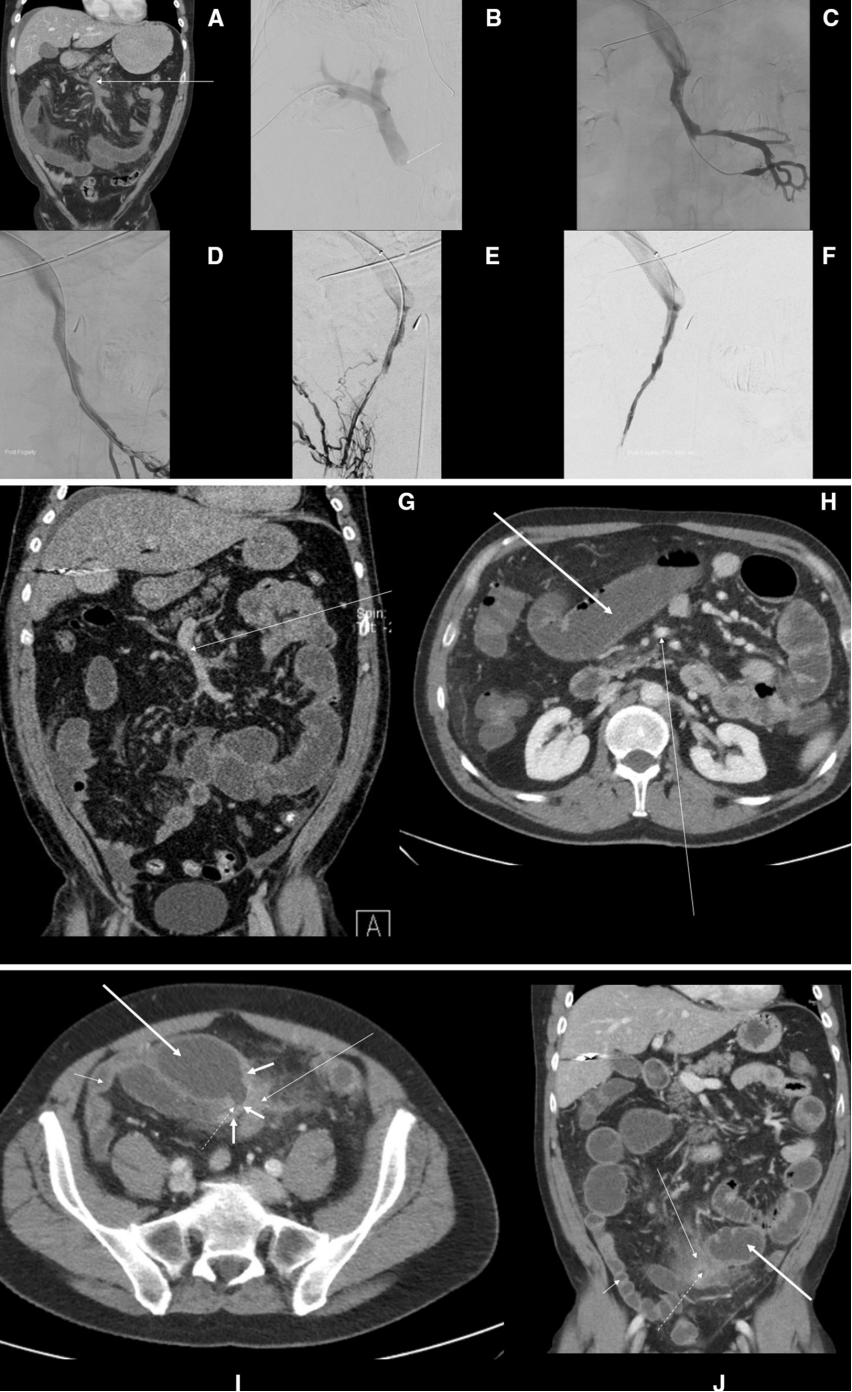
Endovascular therapy of mesenteric venous thrombosis after failure of anticoagulation treatment. A 53-year-old man with history of 3 months of anticoagulation treatment for deep venous thrombosis in the lower leg and Factor V Leiden mutation in the homozygous form. The patient fell ill with acute abdominal pain, and CT diagnosed an extensive MVT (a, arrow). He underwent transhepatic puncture and access to the portal vein. Venography showed total occlusion of the superior mesenteric vein (SMV) (b, arrow). Mechanical thrombectomy with an AngioJet® device (MEDRAD, Warrendale, Pennsylvania, USA) and endovascular Fogarty catheter thrombectomy were carried out, followed by thrombolysis with recombinant tissue plasminogen activator (rtPA) into the branches of the SMV and superior mesenteric artery. After a total dose of 25 mg rtPA over 25 h, improved flow in the SMV was noted. Endovascular rethrombectomy with a Fogarty catheter was performed owing to residual clots in the SMV branches (c–f). CT venography before discharge showed no signs of thrombus within the SMV and the proximal parts of the major venous branches (g, arrow). The patient did not recover fully and was readmitted after 3 months with symptoms of bowel obstruction. CT venography showed fully patent SMV (h, arrow), but severe localized fibrosis in the small bowel wall (i, thick short arrows) and adjacent mesenteric fat (i, j, thin long arrow) causing a bowel stricture. Note the narrow bowel lumen at the stricture (i, j, interrupted line). There is a prestenotic bowel dilatation (h, i, j, thick long arrow) and a poststenotic normalized bowel (i, j, thin short arrow). The patient underwent immediate bowel resection of the stricture, recovered and is on lifelong vitamin K antagonist medication
